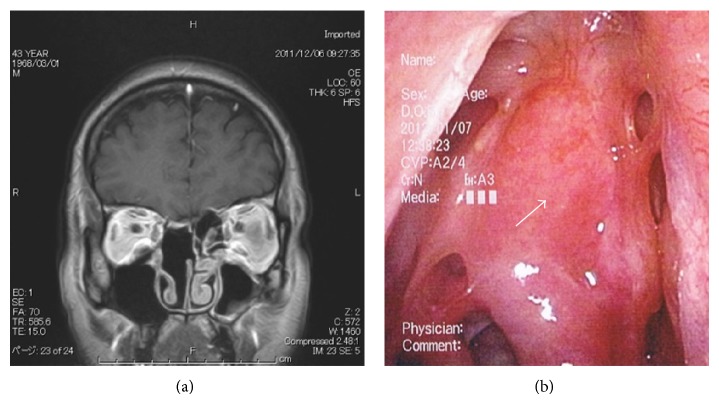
Postoperative MRI and local finding in case 1. (a) A T1-weighted coronal image (gadolinium+) showing no recurring mass. (b) The white arrow shows the pedicled nasal septal flap in the right nasal cavity.
